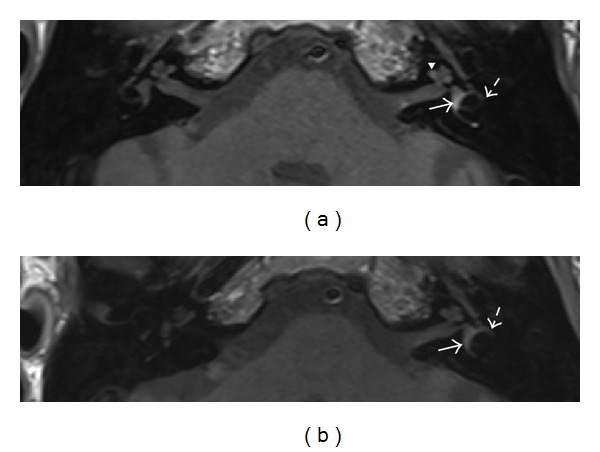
(a) T1 weighted native MRI of the petrous bone showing hemorrhage in the vestibule (arrow) and in the semicircular canals (partly shown, striped arrow) and slightly in the basal (not shown) and second turn (arrowhead) of the cochlea on the left. (b) 3-month follow-up shows clear resolution of the hemorrhage. Only slight residual blood is visible in the vestibulum (arrow) and in the semicircular canals (partly shown, striped arrow).
